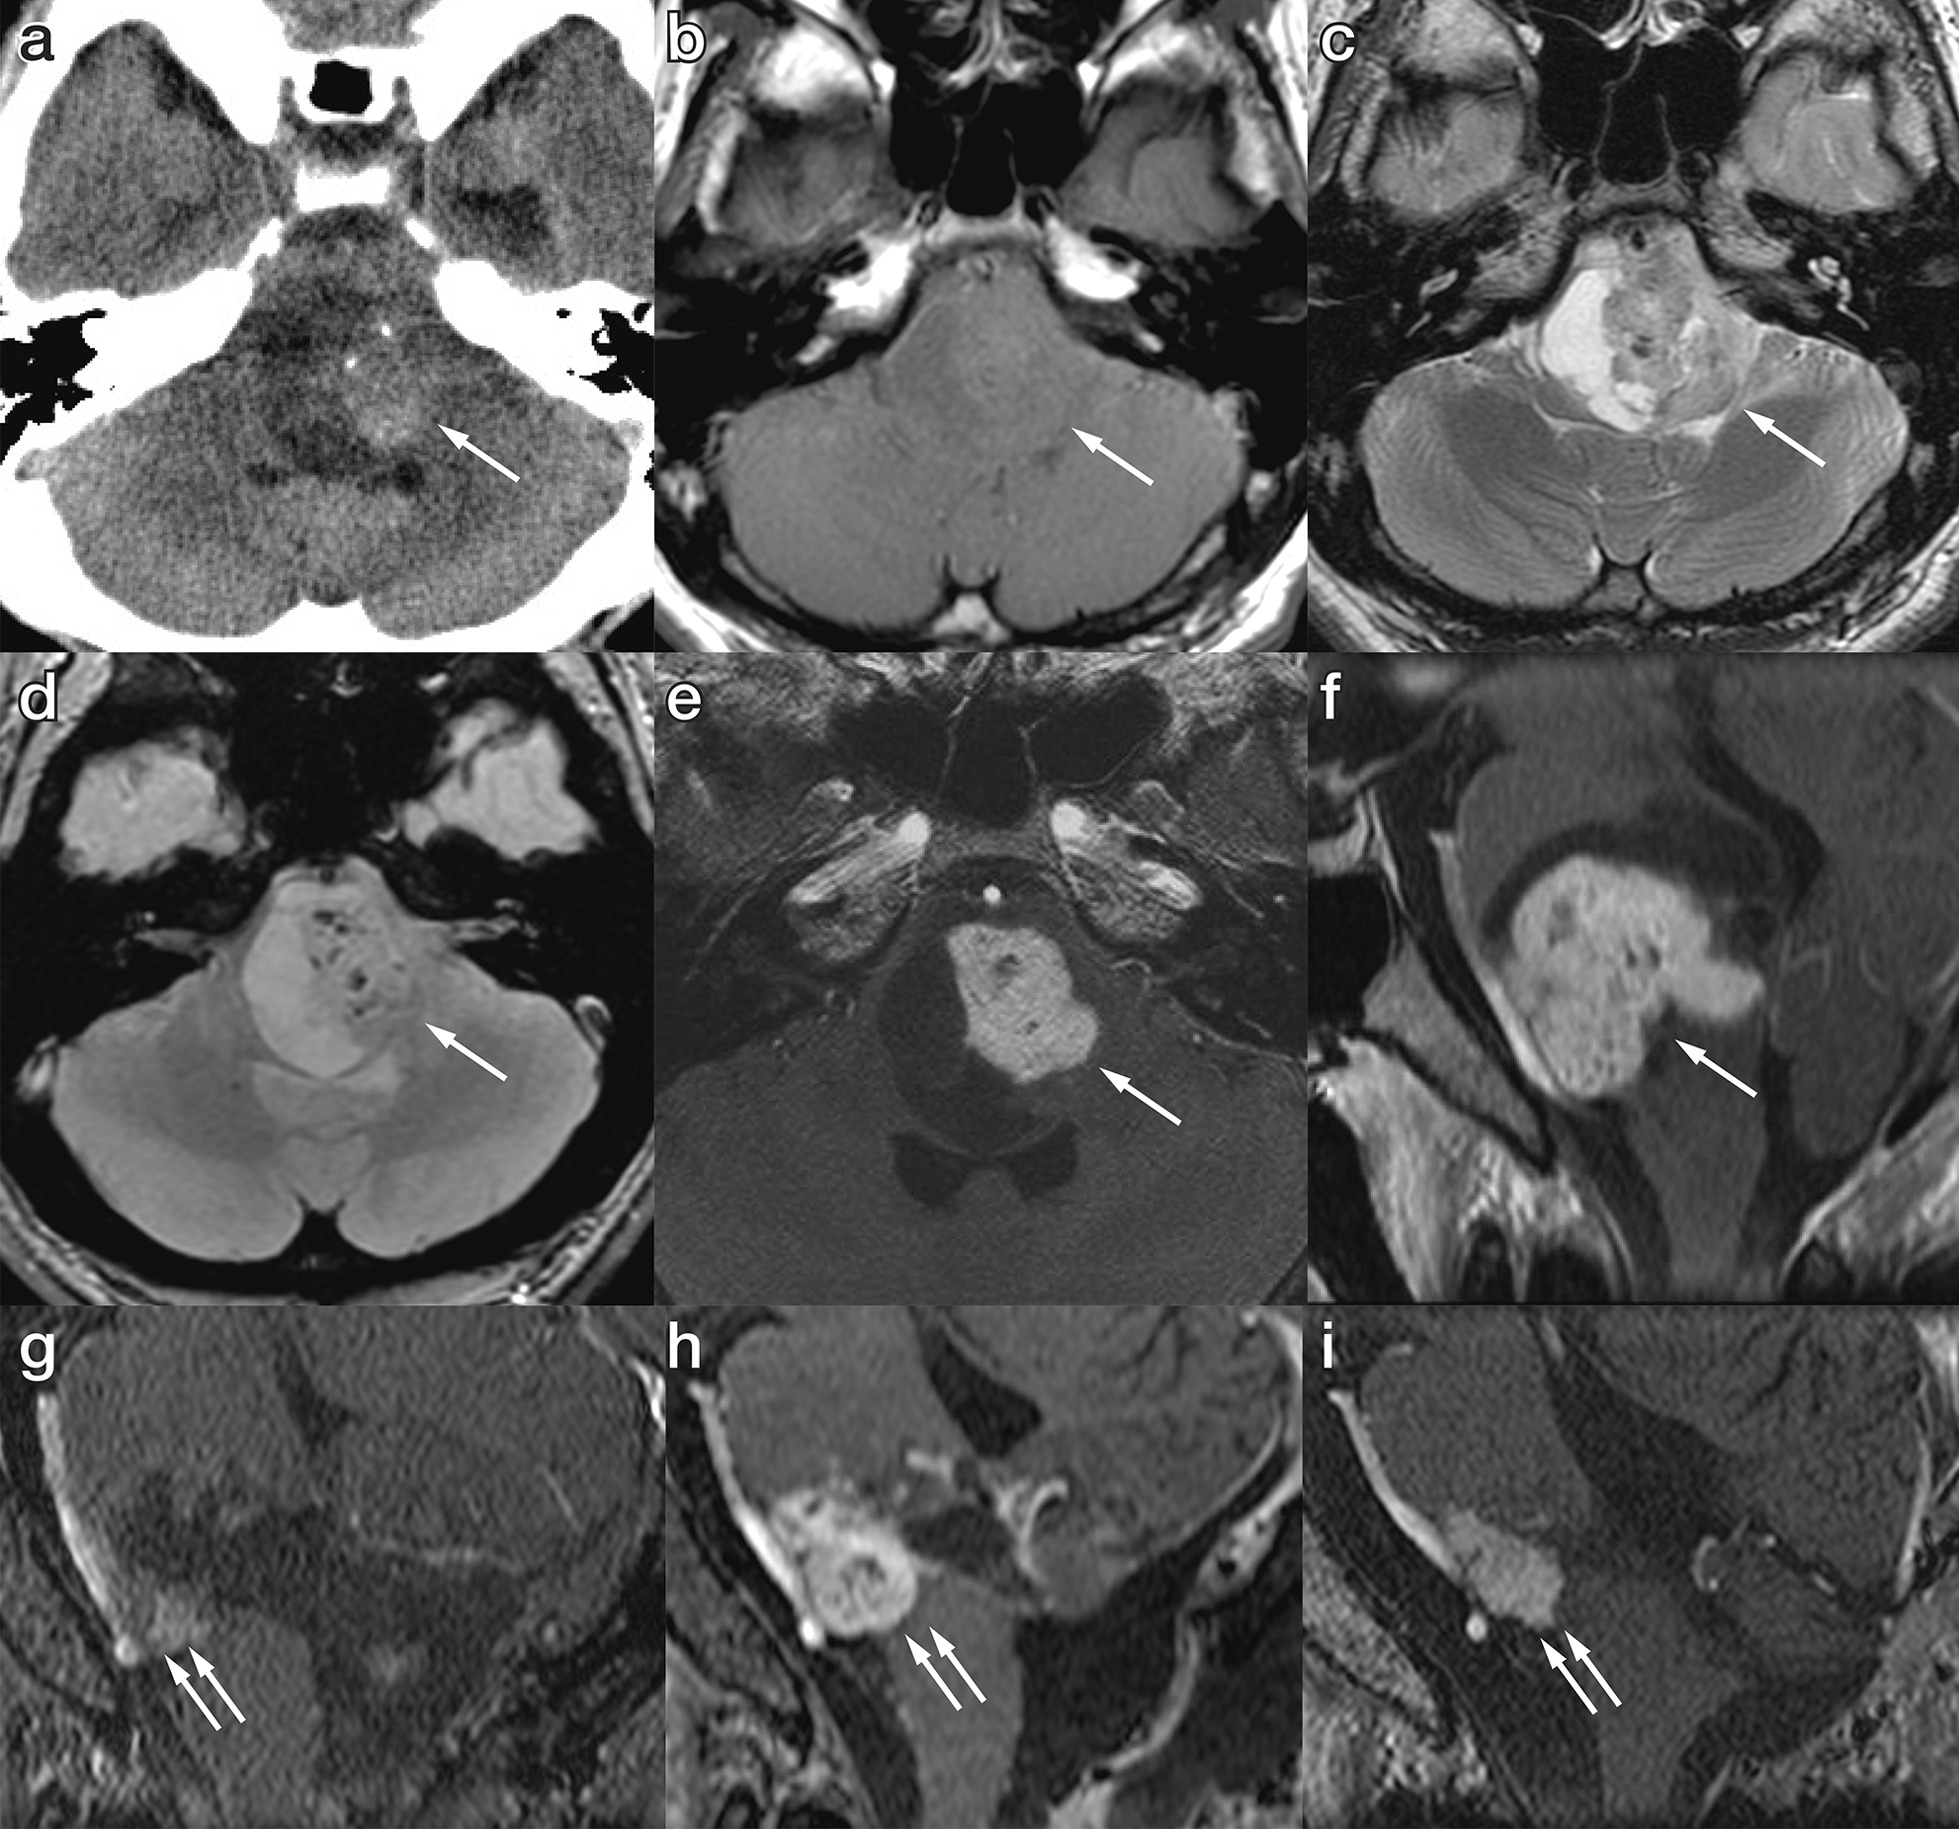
Computed tomography (CT) and magnetic resonance imaging (MRI) performed on admission. a Non-contrast CT, b T1-weighted imaging, c T2-weighted imaging, d T2* imaging, e gadolinium-enhanced T1 imaging (axial), and f sagittal reconstructed imaging. The tumor comprised a solid and a cystic component. The solid part was slightly calcified. The posterior wall of the cyst was adjacent to the floor of the fourth ventricle. g Gadolinium-enhanced T1-weighted imaging (Gd-T1WI) in the sagittal plane was performed on postoperative day 1, which showed the residual tumor (double arrows). h Gd-T1WI in the sagittal plane performed on postoperative day 43 showed marked enlargement of the gadolinium-enhanced solid component. i Gd-T1WI in the same slice as (g, h), which was obtained 4 years after tumor resection and irradiation. Slight shrinkage of the solid component was observed. The arrow indicates the tumor in (a–f), and the double arrows point to the postoperative residual tumor in (g–h)
